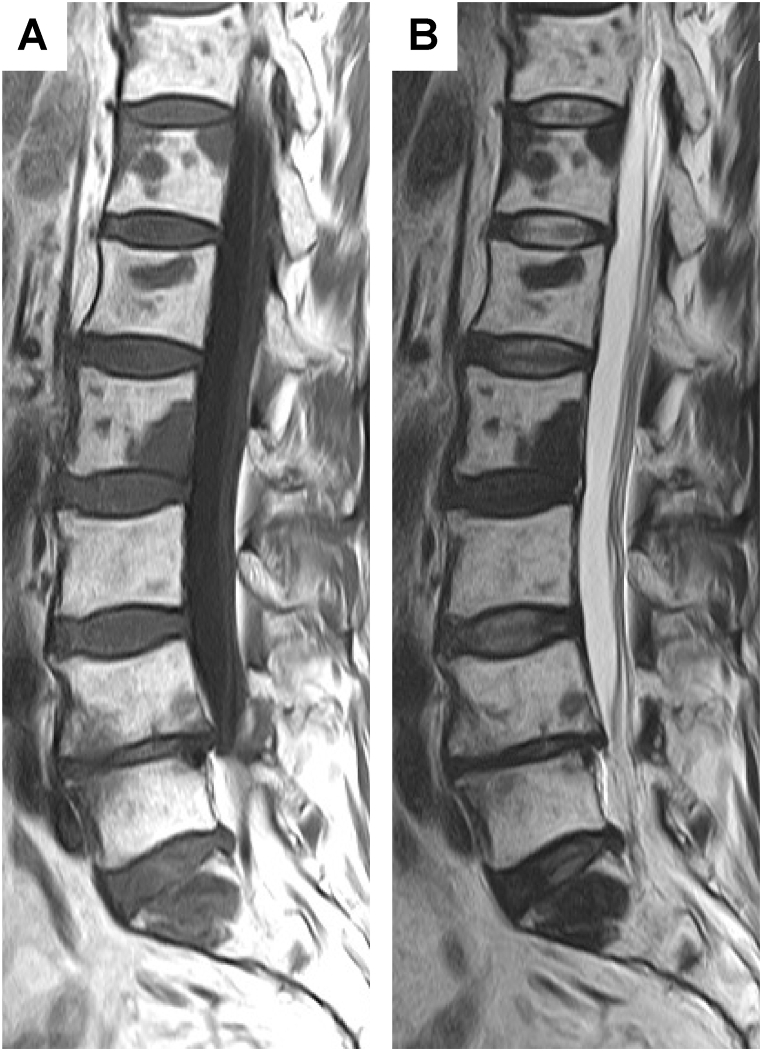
Sagittal magnetic resonance image of the thoracic spine shows multiple vertebral body lesions. T1-weighted (A) and T2-weighted (B) images show multiple hypointense masses.
